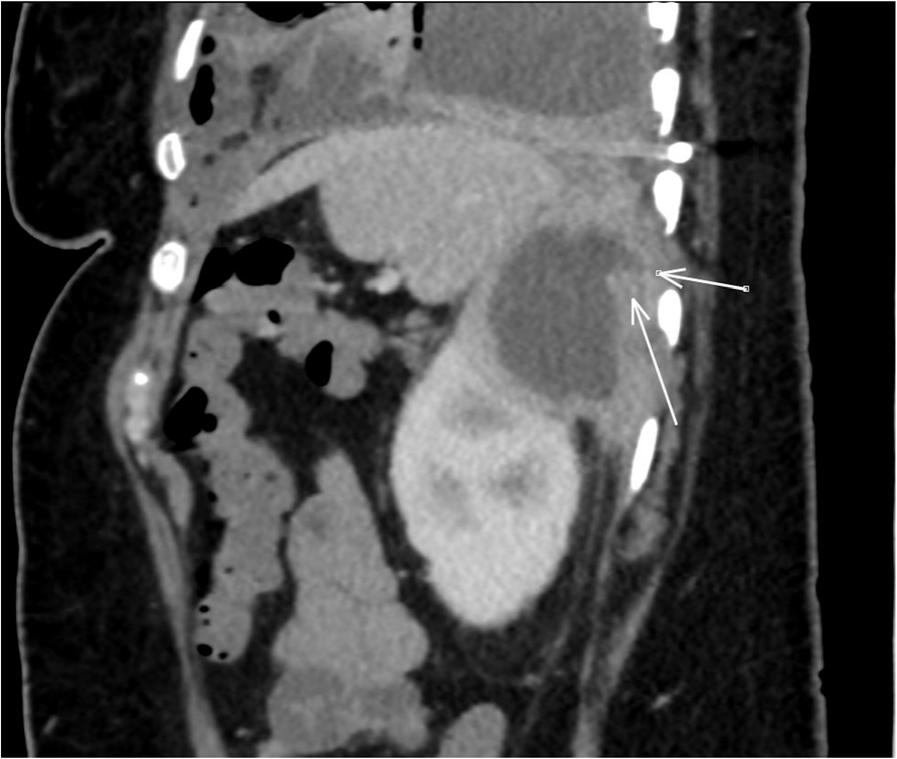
Computed Tomography results showing a sagittal cross section of the patient’s abdomen with the white arrows indicating the renal cyst and tract extending posteriorly to the diaphragm
